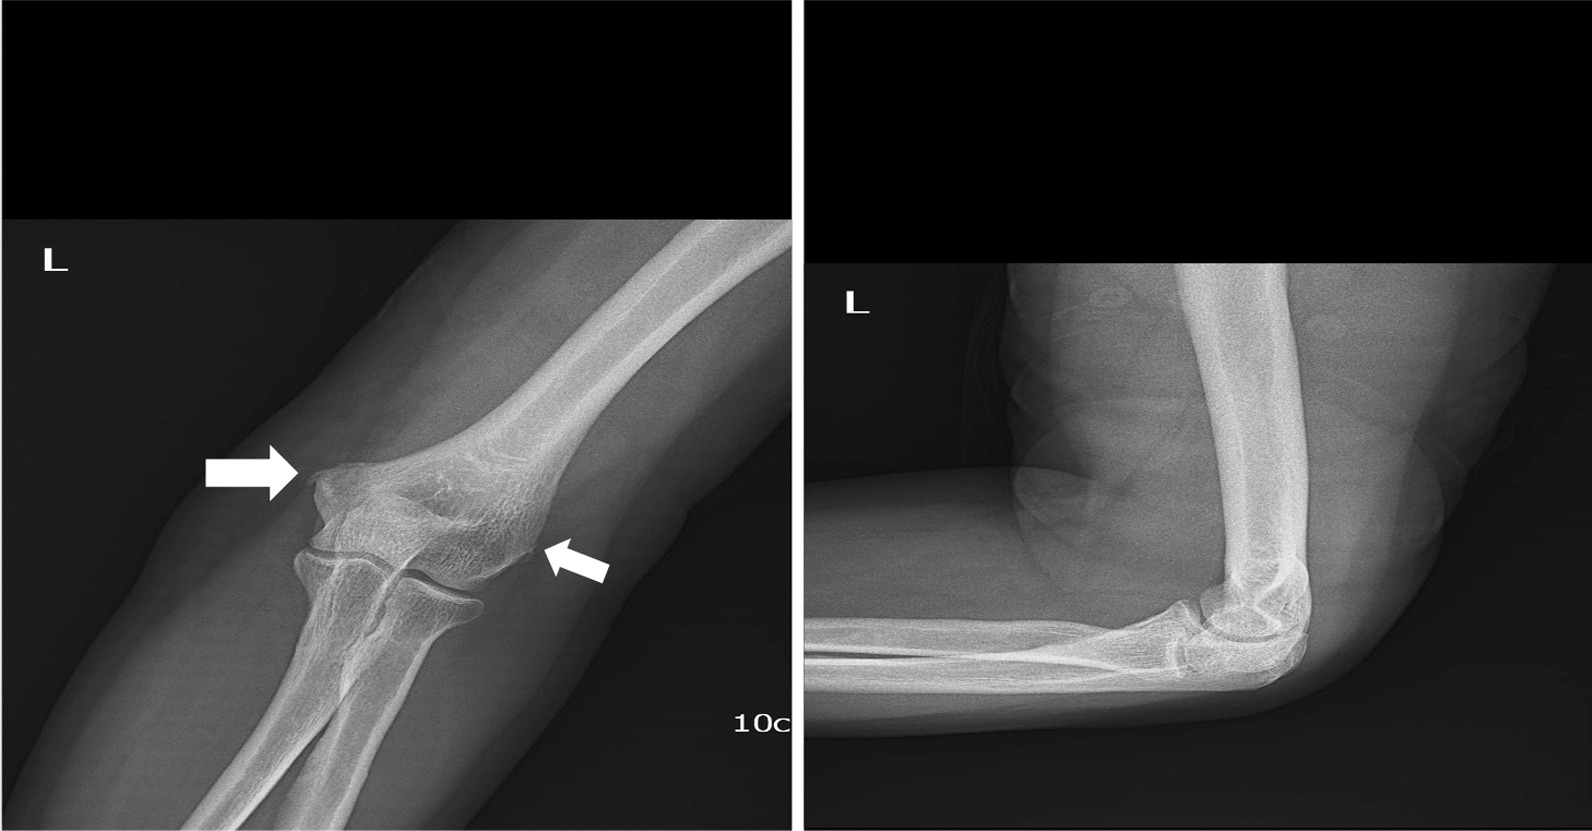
Anterior–posterior and lateral view radiographs of the left elbow. The imaging shows the presence of a small calcification in the soft tissues near both epicondyles mainly in the lateral epicondyle (arrow)
